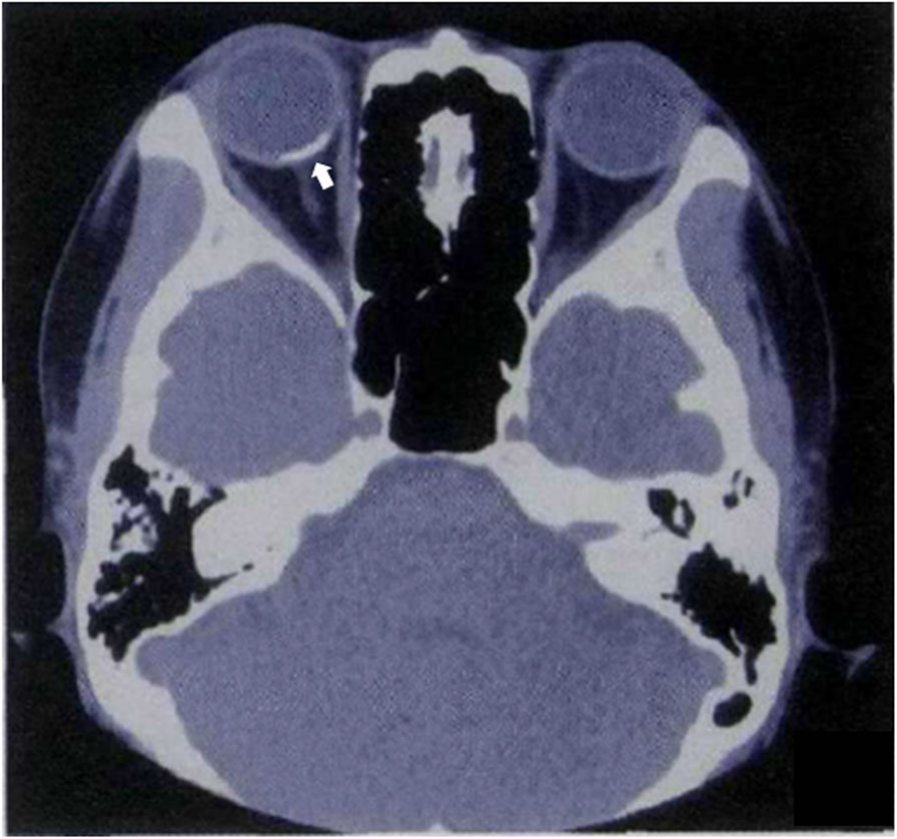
CT demonstrated a hyperdense choroidal plaque with the same density as bone typically (white arrow)
